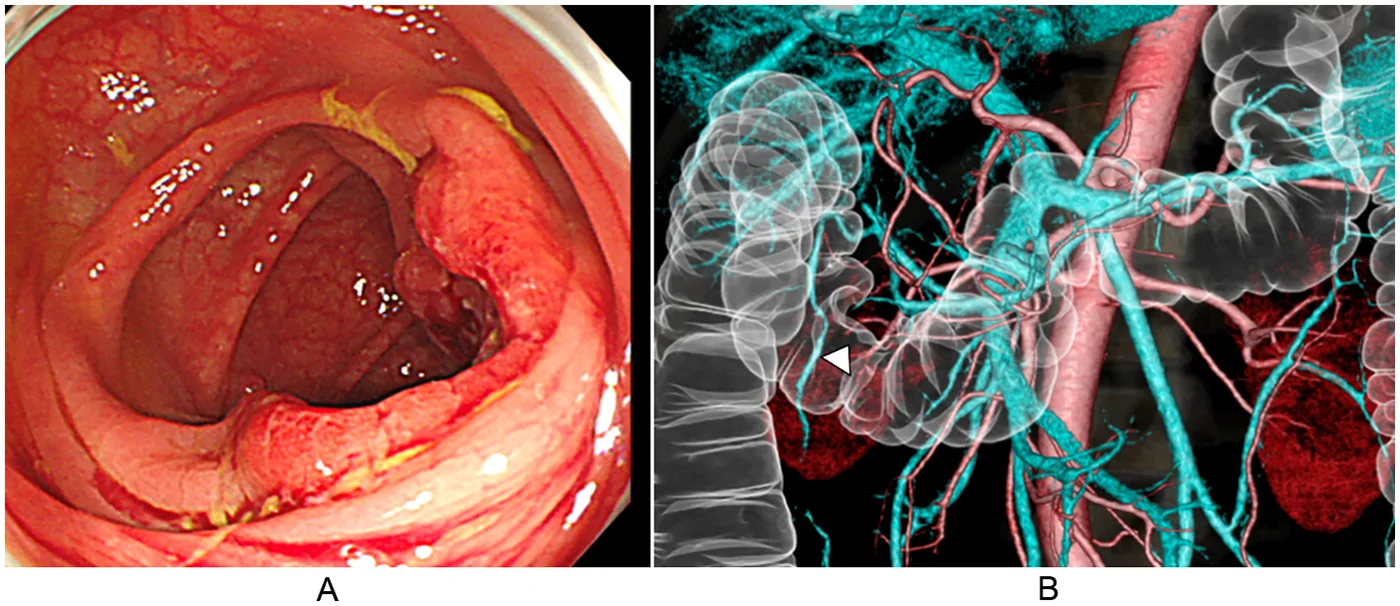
Endoscopic and radiological findings of the tumor. (A) Colonoscopy revealed a semicircumferential, circumscribed ulcerative lesion measuring 4 cm in diameter in the proximal transverse colon, causing significant luminal narrowing. (B) Three-dimensional (3D) reconstructed CT image demonstrating the tumor in the proximal transverse colon (white arrowhead).
